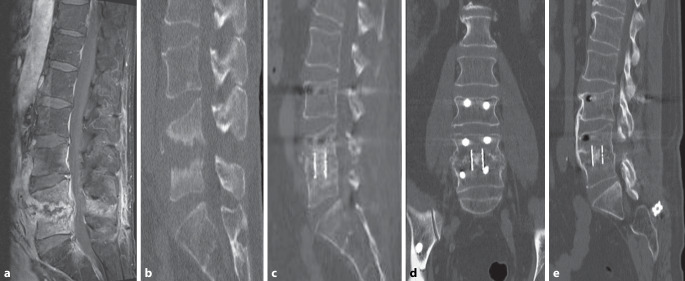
A 60-year-old patient diagnosed with lumbar pyogenic spondylodiscitis at L4–5 underwent posterior debridement and interbody fusion, with a gentamicin-loaded antibiotic carrier applied intraoperatively. a,b Preoperative MRI and CT scans demonstrate destruction of the L4–5 disc space and adjacent vertebral bodies. c,d At 3 months postoperatively, the patient showed Bridwell grade 3 fusion. e At 16 months postoperatively, CT scans reveal complete bony fusion at the treated level
